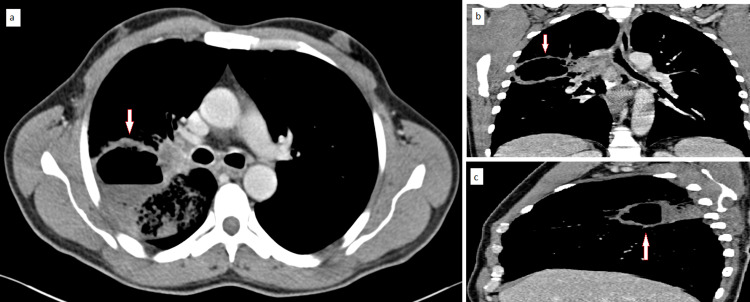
Axial (a), coronal (b), and sagittal (c) computed tomographic scans showing a large cavitating heterogeneous mass of the right upper lobe (arrow) with lobulated margins in the lower lobe of the right lung measuring approximately 8.7 × 8.2 × 7.4 cm.
